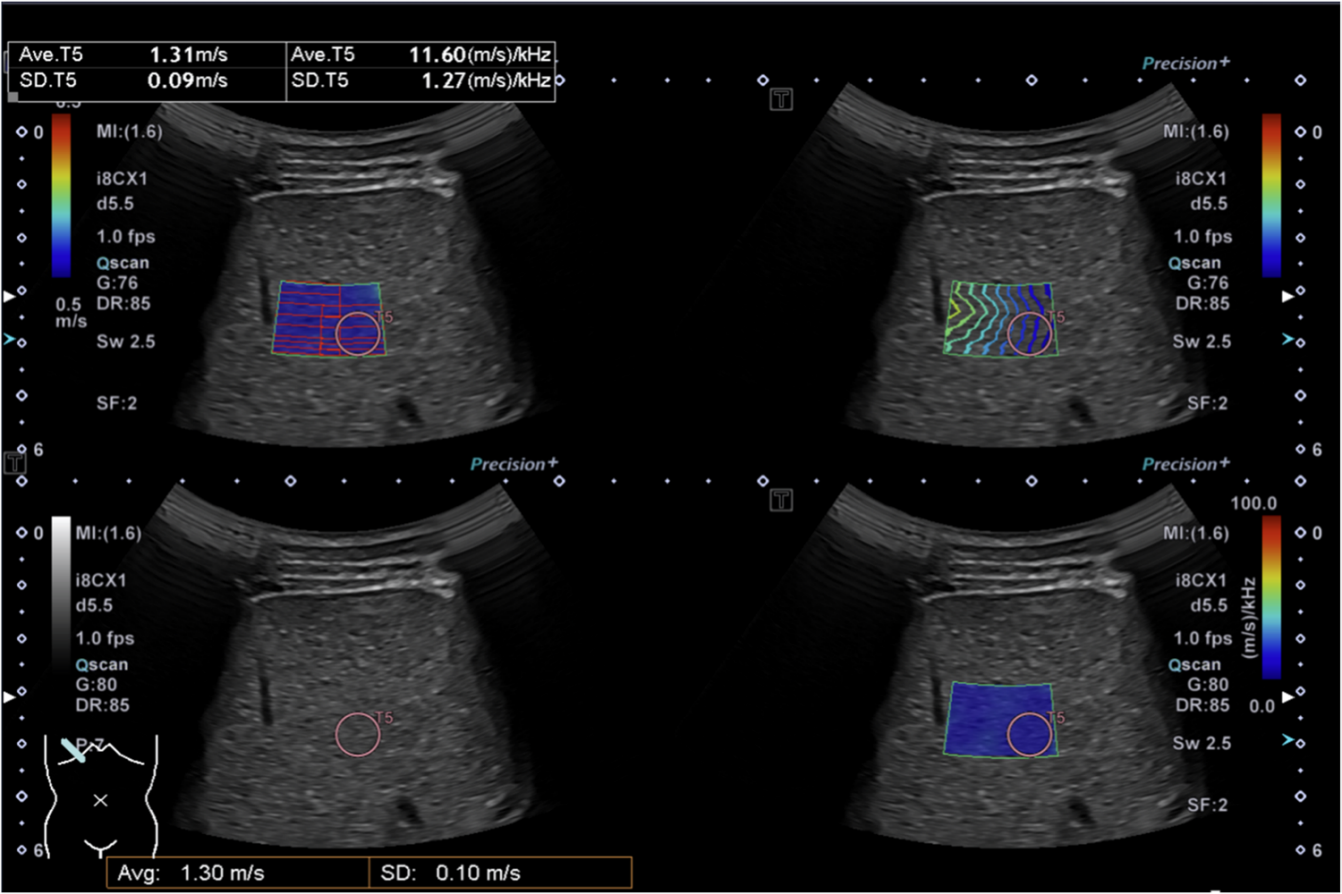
Representative quad view measurement number five out of 12 (liver ultrasound in the transverse intercostal plane) in a 14-year-old healthy boy with a shear wave elasticity of 1.31 m/s and a shear wave dispersion of 11.60 (m/s)/kHz
